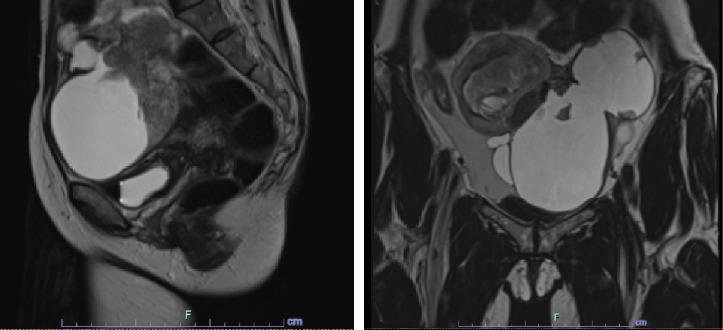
Magnetic resonance image showing an enlarged and leiomyomatous uterus measuring approximately 7.5 × 0.6 × 5.3 cm. There is also a large pelvic mass that is cystic and solid appearing measuring approximately 9.7 × 15.8 × 12.2 cm. The left and right ovaries cannot be distinguished on this exam.
